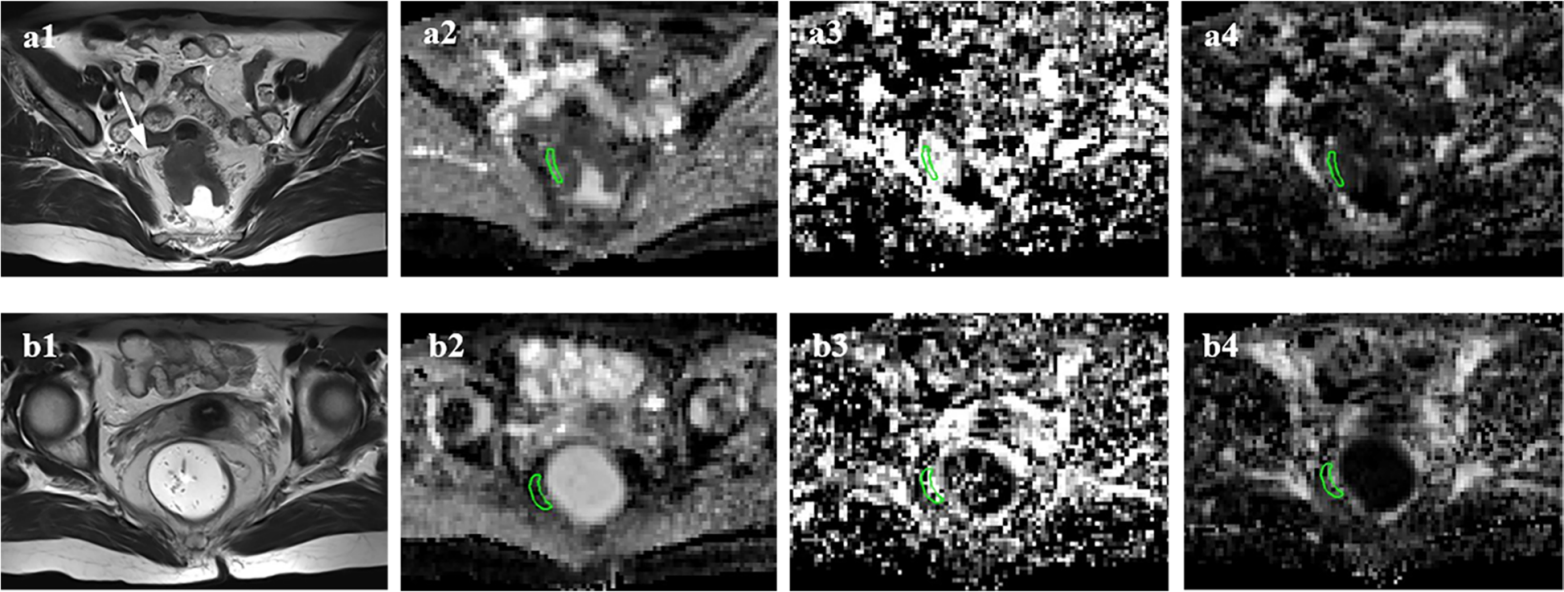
Measurement of IVIM parameters in the mesorectum in patient with positive mrCRM. Axial T2-weighted imaging shows a tumour lying within 1 mm of the mesorectal fascia (a1, arrow). The ROIs were delineated in the D (a2), D* (a3) and *f* (a4) maps in the MAT. And the values of D, D* and *f* were 1.38 × 10^–3^ mm^2^/s, 22.95 × 10^–3^ mm^2^/s and 0.21, respectively. The ROIs in the MDT were more than 10 mm away from the largest tumour cross-section (b1). The corresponding IVIM maps were showed in b2, b3 and b4, and the values of D, D* and *f* were 0.54 × 10^–3^ mm^2^/s, 11.47 × 10^–3^ mm^2^/s and 0.29, respectively
